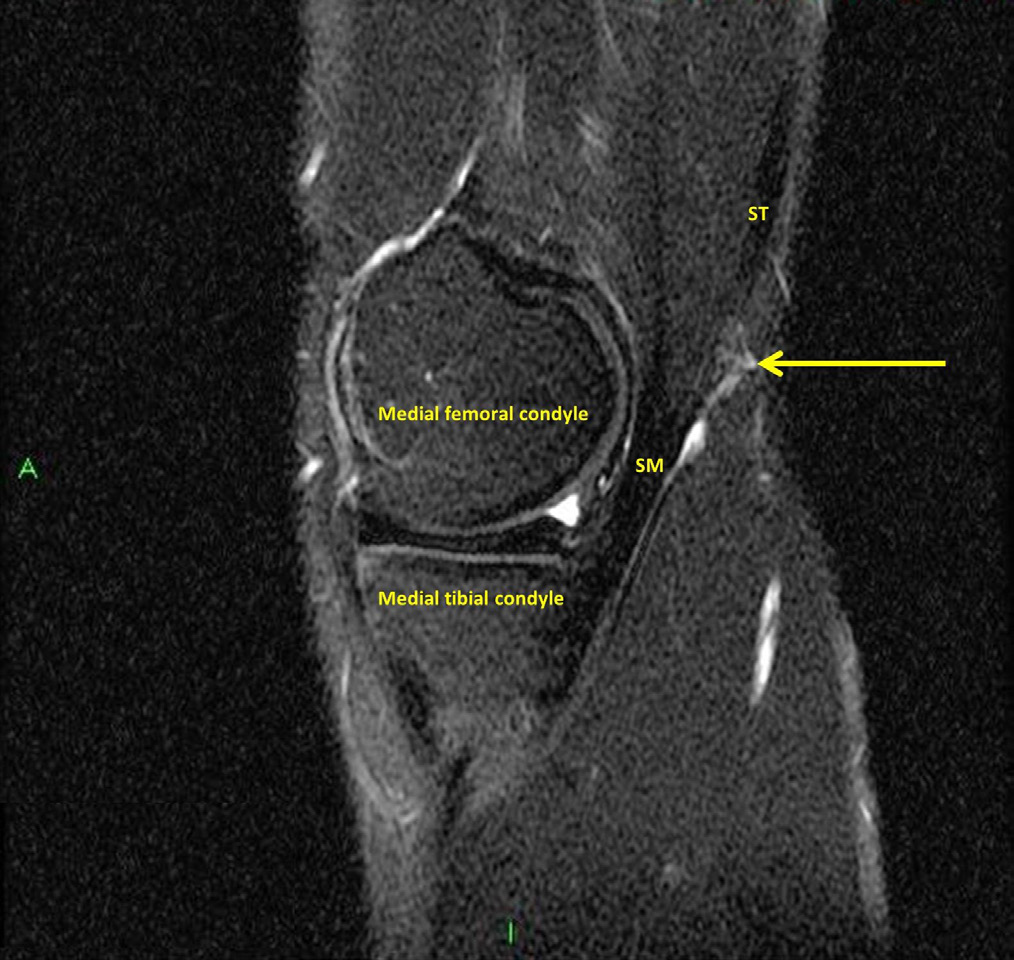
Sagittal proton density fat saturated MRI image demonstrating the intact
